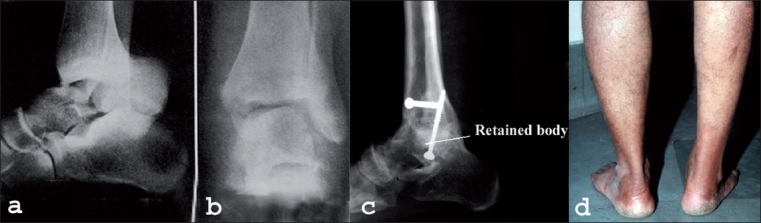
Anteroposterior (a) and Lateral (b) X-ray of ankle and foot of a 30-year-old man having 42 days' old injury shows fracture dislocation of talus. Lateral X-ray (c) nine year of follow-up shows solid fusion. Talar body is incorporated into the tibia, leading to normal hind foot alignment. The clinical photograph (d) at 9 years follow-up shows excellent appearance
